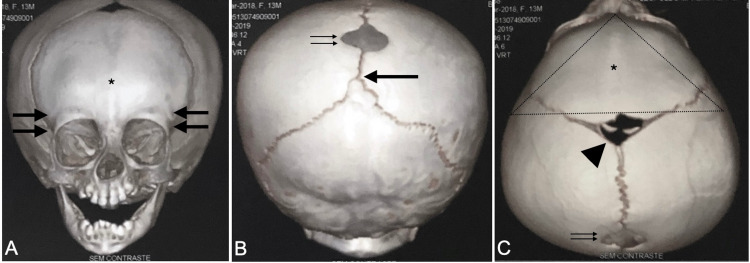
The computerized tomography scan of the skull revealing the cranium bifidum occultum associated with trigonocephaly This 3D reconstruction of a CT scan of the skull depicts trigonocephaly associated with a midline defect in the parietal bone. In the anterior view of skull, a fused metopic suture (*) and bitemporal narrowing (double thick black arrows) can be observed (A). The posterior view displays a closed posterior fontanelle (thick black arrow) and a bone defect (double thin black arrows), corresponding to cranium bifidum (B). The superior view depicts a trigonocephalic shape of the skull (dot point triangle) with an open anterior fontanelle (arrowhead) (C).
